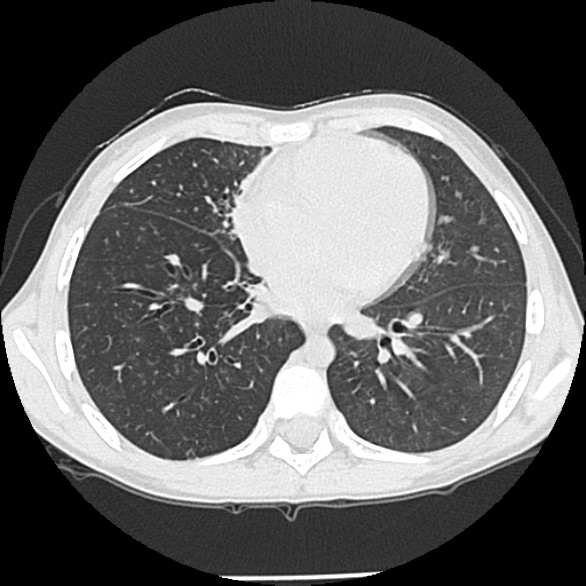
Representative high resolution CT chest slices demonstrating 'mild' bronchiectasis of the right lower lobe with partial collapse of right middle lobe. Bronchiectasis also present in the right middle lobe is not clearly demonstrated here.
